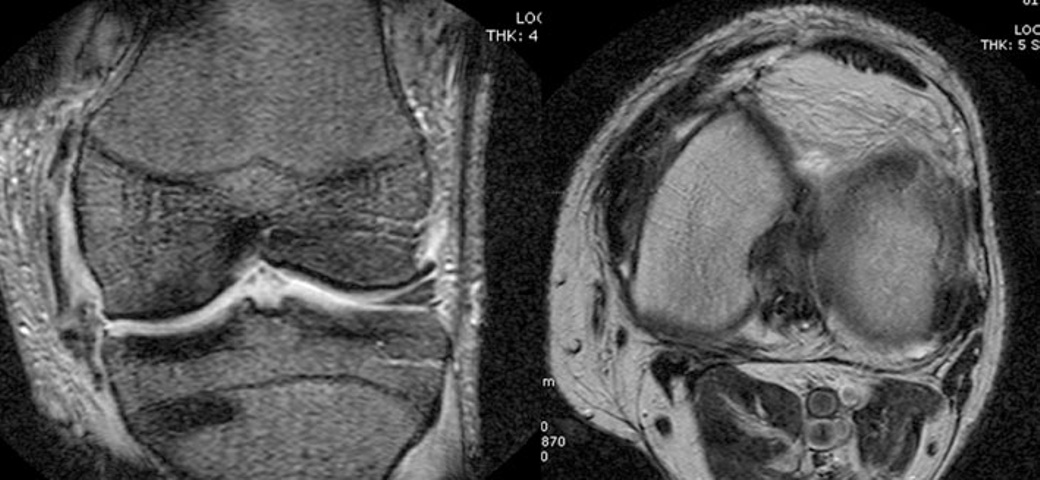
MRI at 6 months showing the healing of the MCL, with residual oedema
